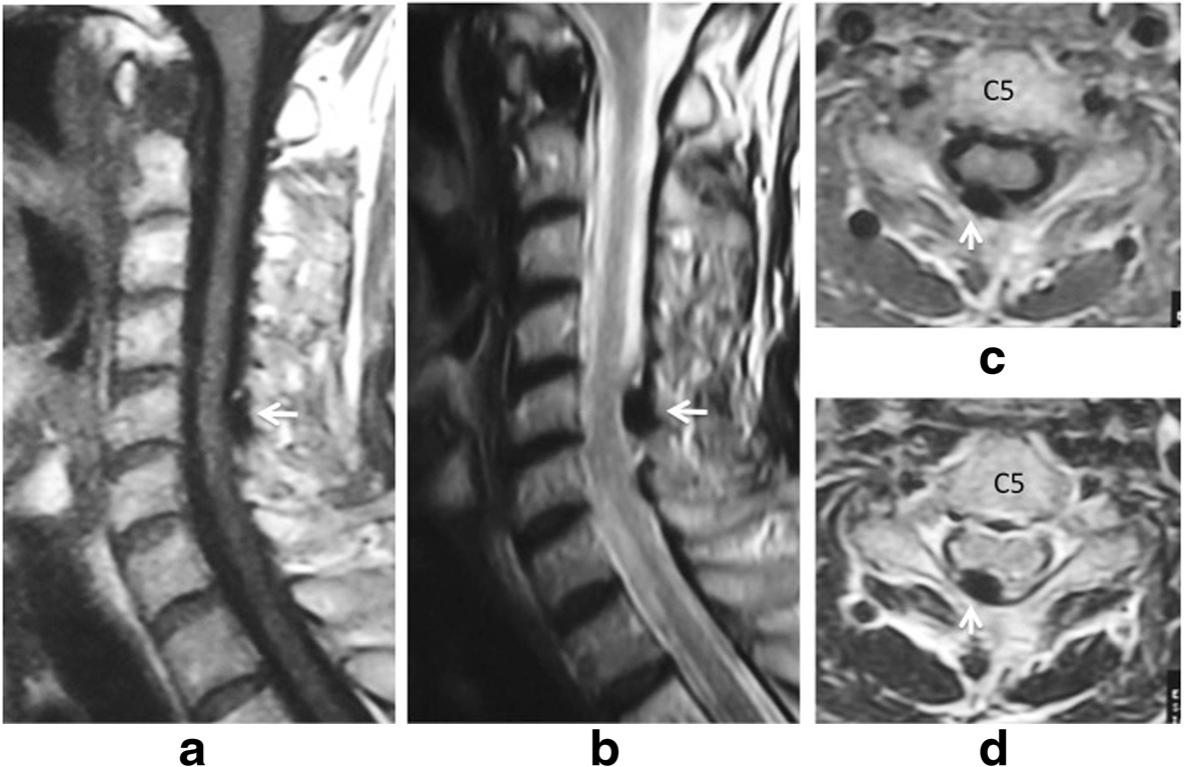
Sagittal T1-weighted imaging (a) and T2-weighted imaging (b), and axial T1-weighted imaging (c) and T2WI (d) magnetic resonance imaging on initial admission. Low signal intensity (*white arrow*) on both T1-weighted imaging (a, c) and T2-weighted imaging (b, d) at interlaminar space of C5-C6 and C6-C7 is seen on the sagittal image (a, b) and axial image (c, d)
